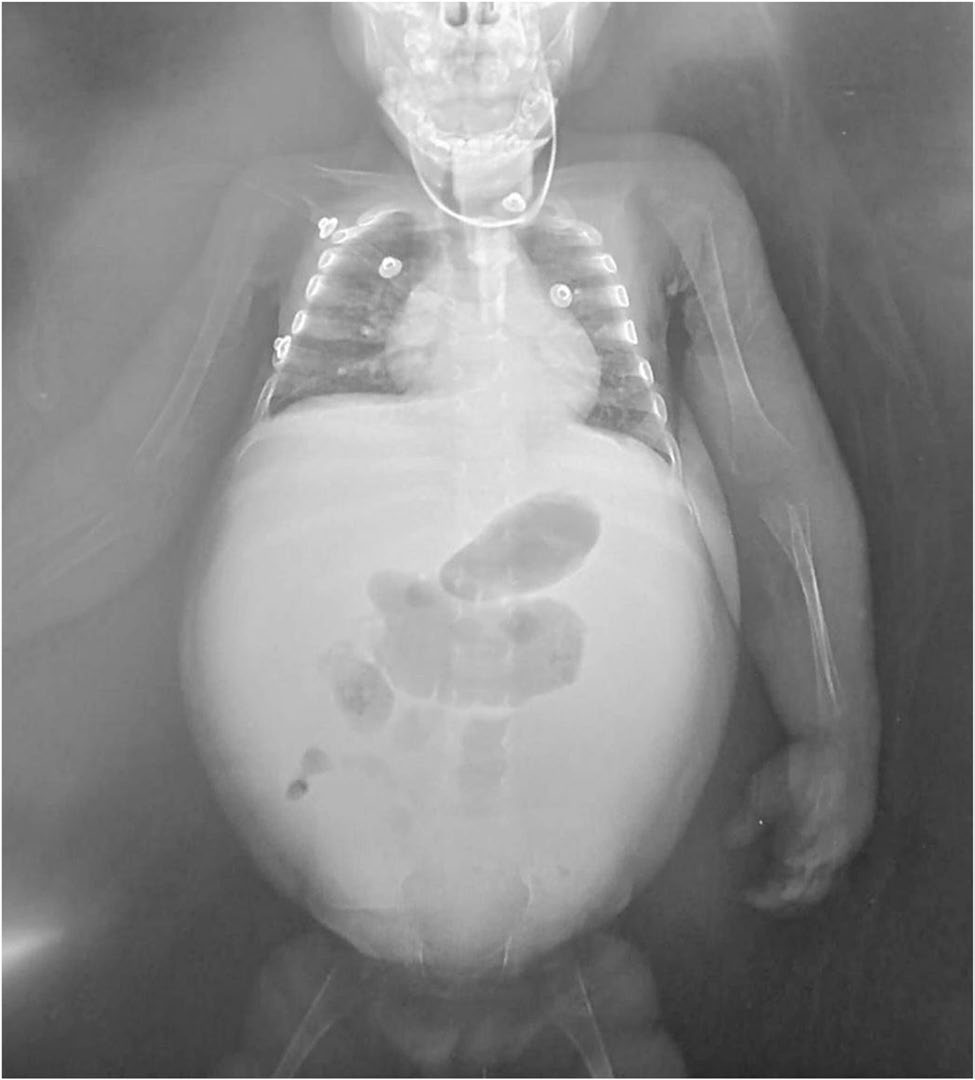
Abdominal X-ray picture showing abdominal distension with dilated bowel loops
